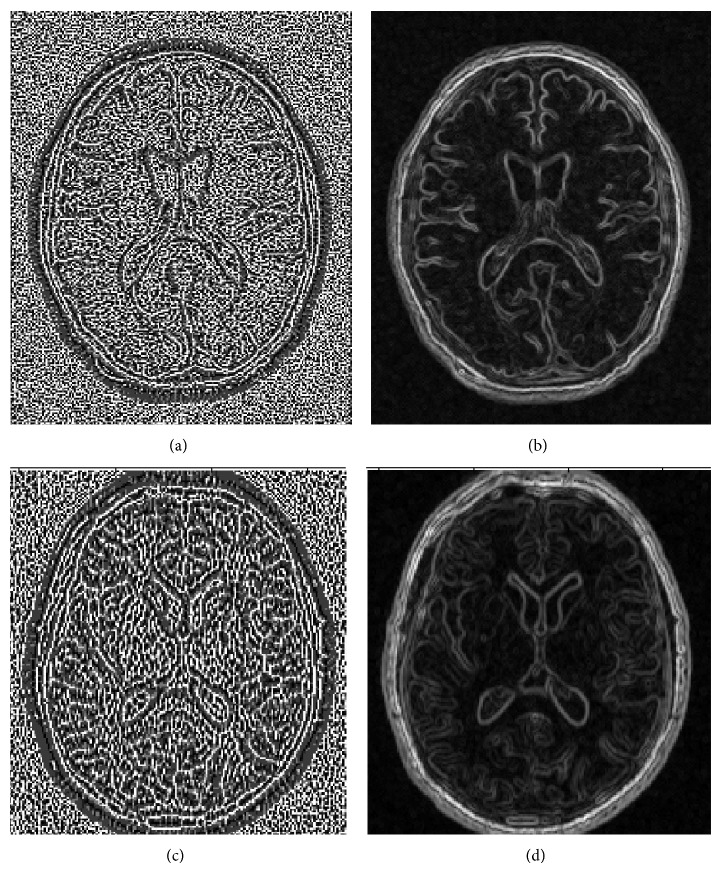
Overview of LBP texture- and contrast-valued images based on the FLAIR and T1 images. (a) in the top left corner shows an example of an LBP-valued image calculated from a FLAIR MR image. (b) in the top right corner shows a contrast-valued image calculated from FLAIR MRI. (c) in the bottom left corner shows an LBP-valued image calculated from a T1 image. (d) in the bottom right corner shows a contrast-valued image calculated from a T1 MR image.
